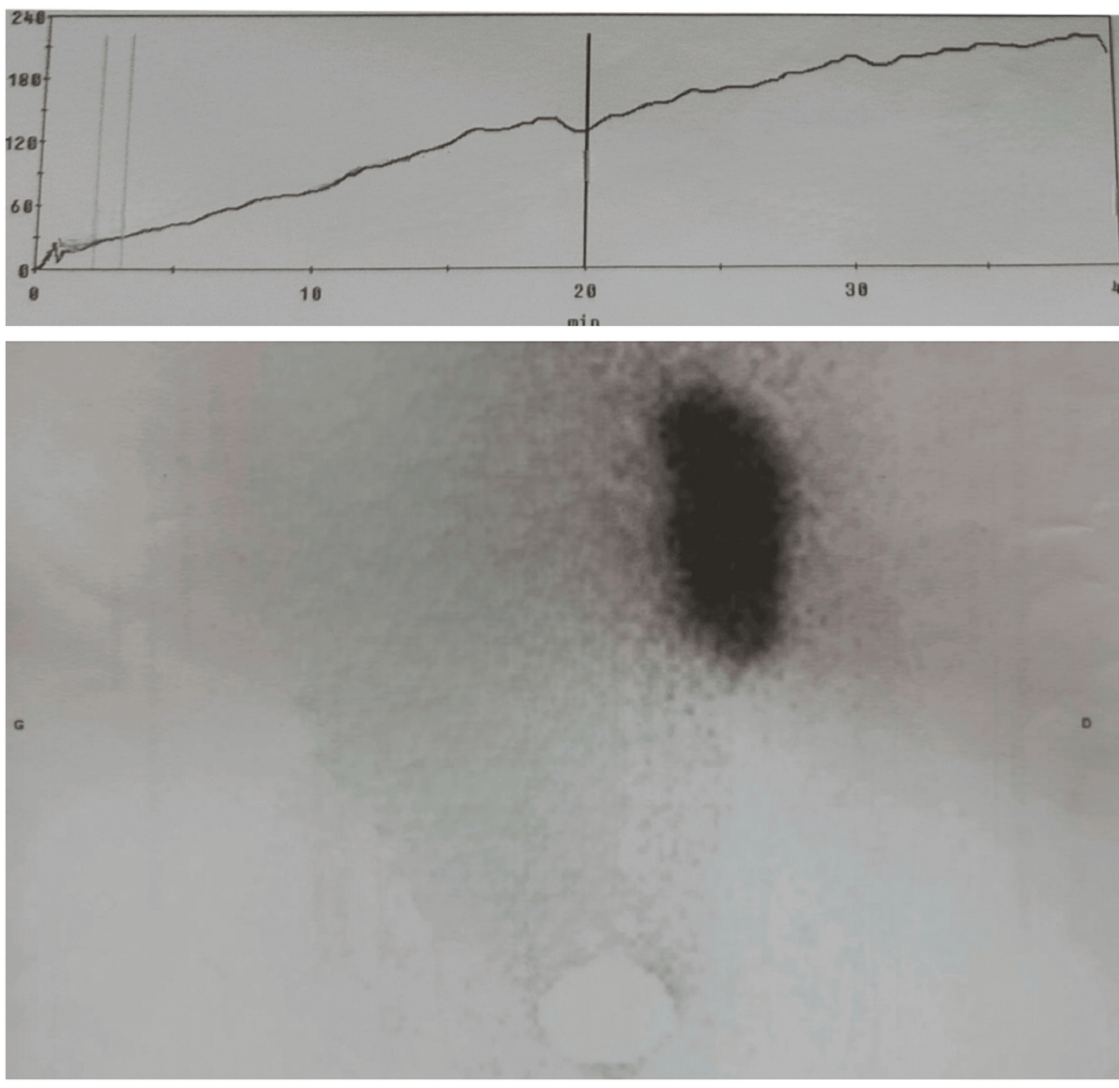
MAG3 renal scintigraphy revealing impaired renal excretion MAG3: mercaptoacetyltriglycine
